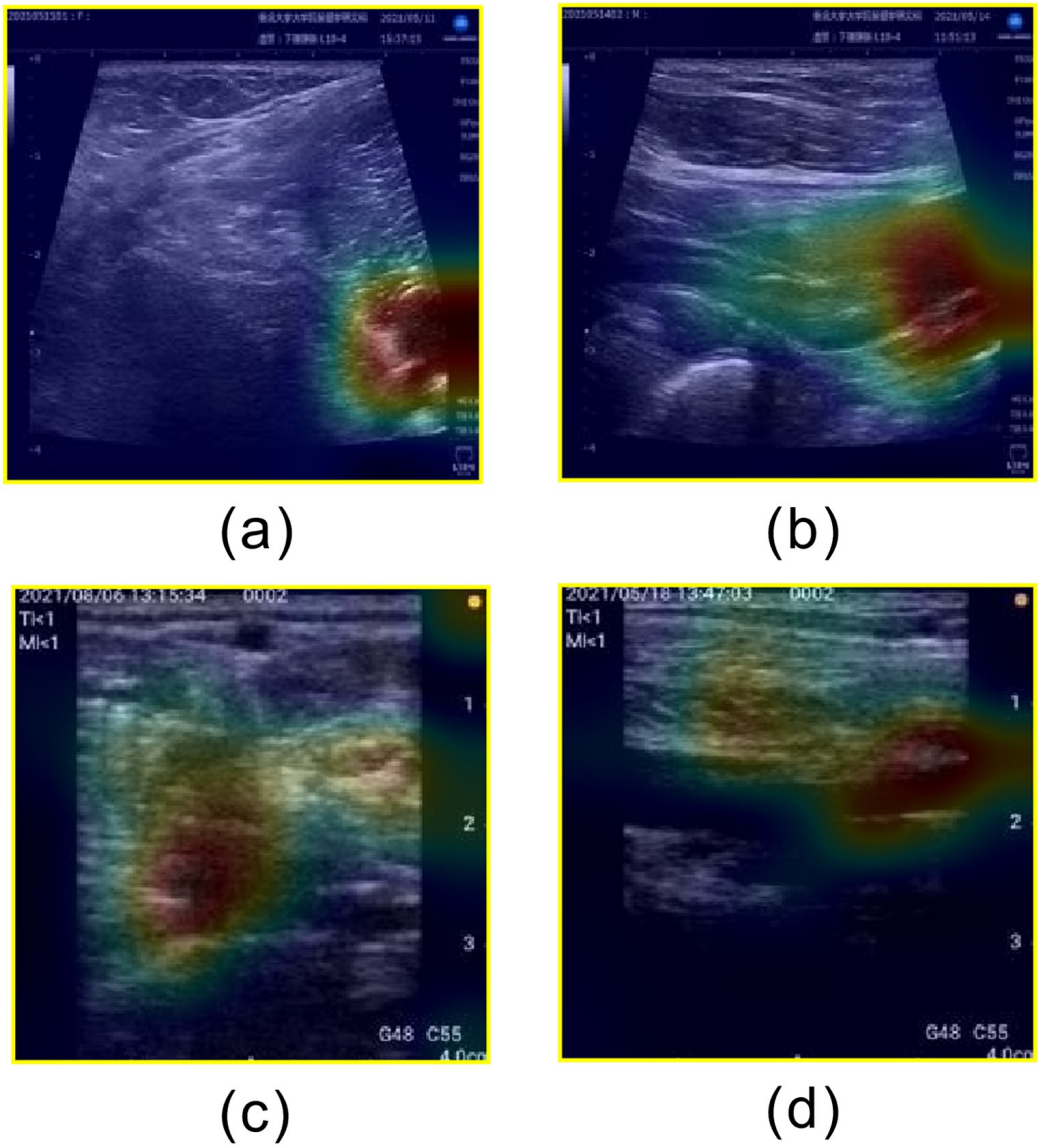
Examples of heatmaps for correctly classified Moderately Satisfactory images. (a) Short axis acquired by stationary ultrasonography equipment, (b) Long axis acquired by stationary ultrasonography equipment, (c) Short axis acquired by portable ultrasonography equipment, and (d) Long axis acquired by portable ultrasonography equipment.
